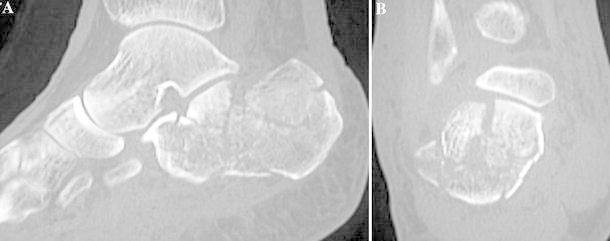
a, b Preoperative CT scan reconstruction showing *vertical* displacement of the thalamic joint surface
